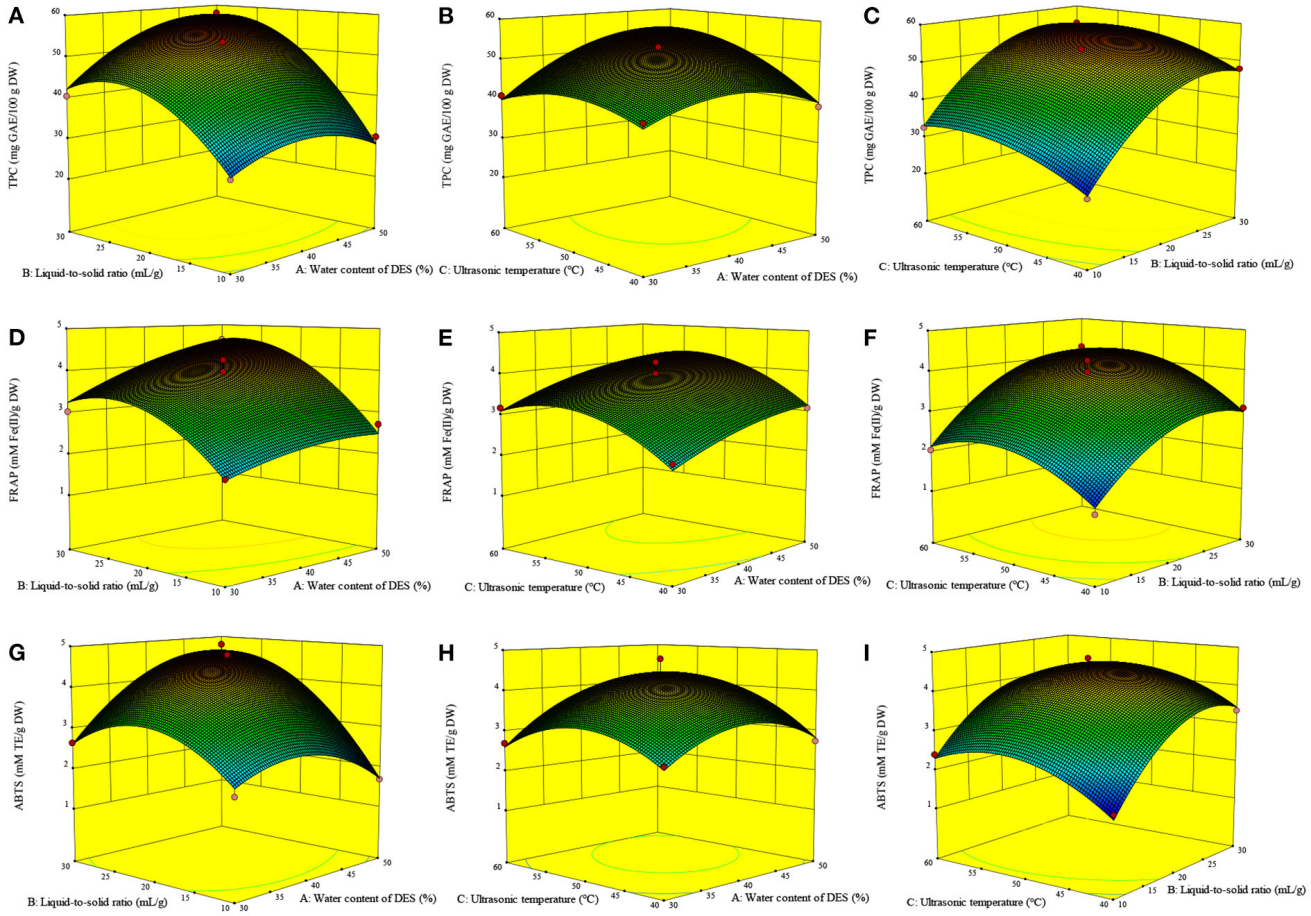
Interactive effects of the variables on the TPC (A–C), FRAP (D–F) and ABTS (G–I) of *Asparagopsis taxiformis* extracts. Interactive effects of water content of DES and liquid-to-solid ratio on TPC (A); Interactive effects of water content of DES and ultrasonic temperature on TPC (B); Interactive effects of liquid-to-solid ratio and ultrasonic temperature on TPC (C); Interactive effects of water content of DES and liquid-to-solid ratio on FRAP (D); Interactive effects of water content of DES and ultrasonic temperature on FRAP (E); Interactive effects of liquid-to-solid ratio and ultrasonic temperature on FRAP (F); Interactive effects of water content of DES and liquid-to-solid ratio on ABTS (G); Interactive effects of water content of DES and ultrasonic temperature on ABTS (H); Interactive effects of liquid-to-solid ratio and ultrasonic temperature on ABTS (I).
